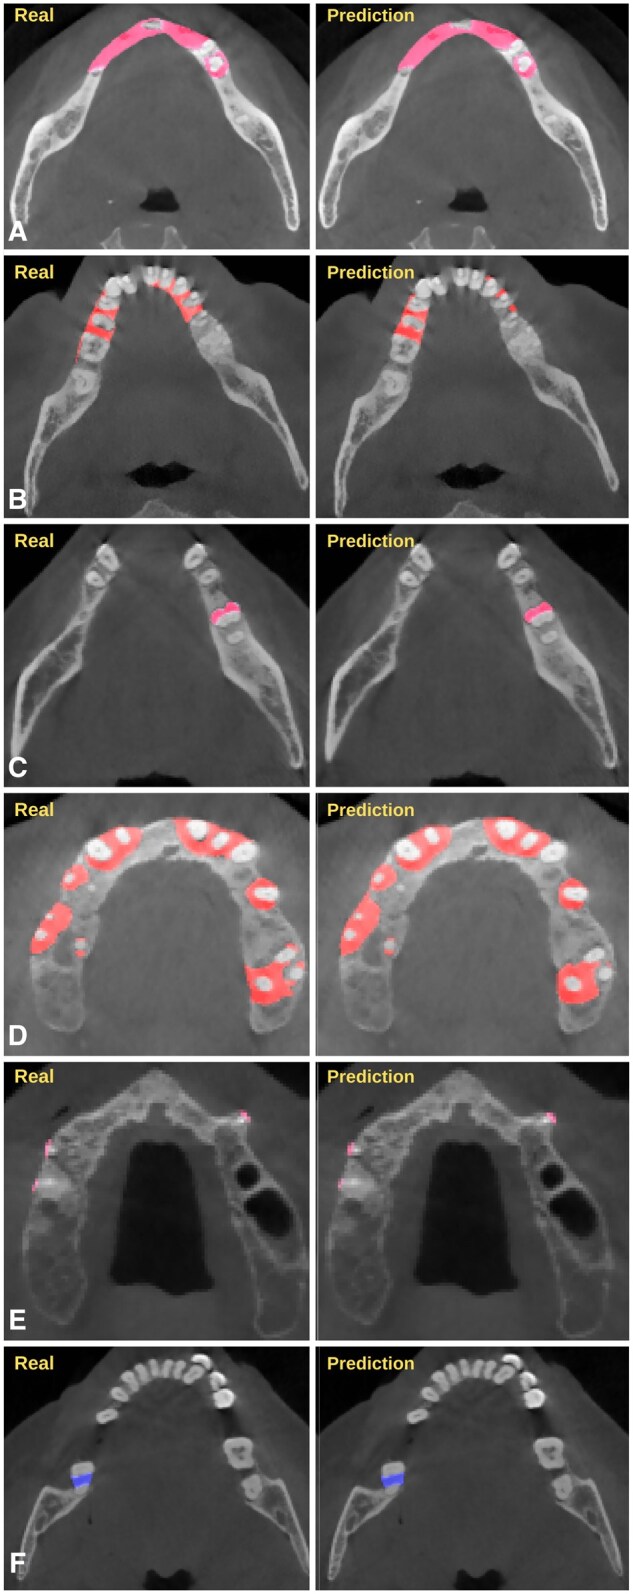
The automatic segmentation of the periodontal problems utilizing the artificial intelligence model in axial cone-beam CT slices. (A) Total alveolar bone loss, (B) supra-bony defects, (C) infra-bony defects, (D) perio-endo lesions, (E) buccal defects, and (F) furcation defects.
